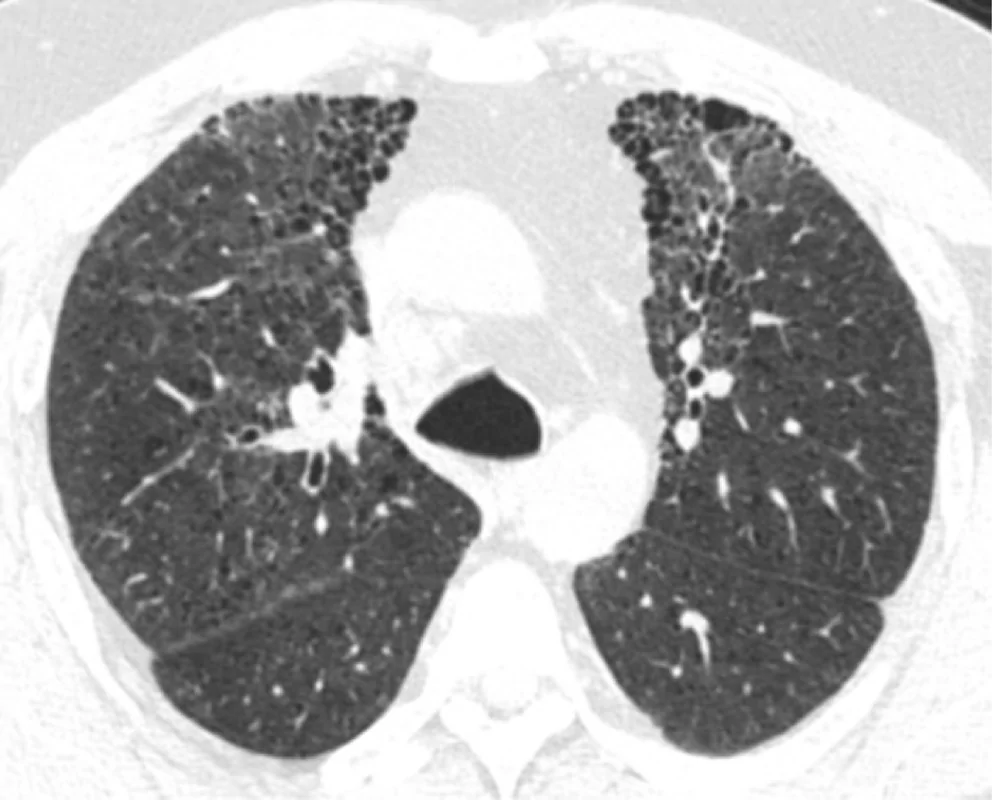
High‐resolution chest CT (lung window) demonstrating upper lobe–predominant subpleural fibrotic interstitial lung disease, characterised by bilateral honeycombing, reticulation and associated traction bronchiectasis/bronchiolectasis.
